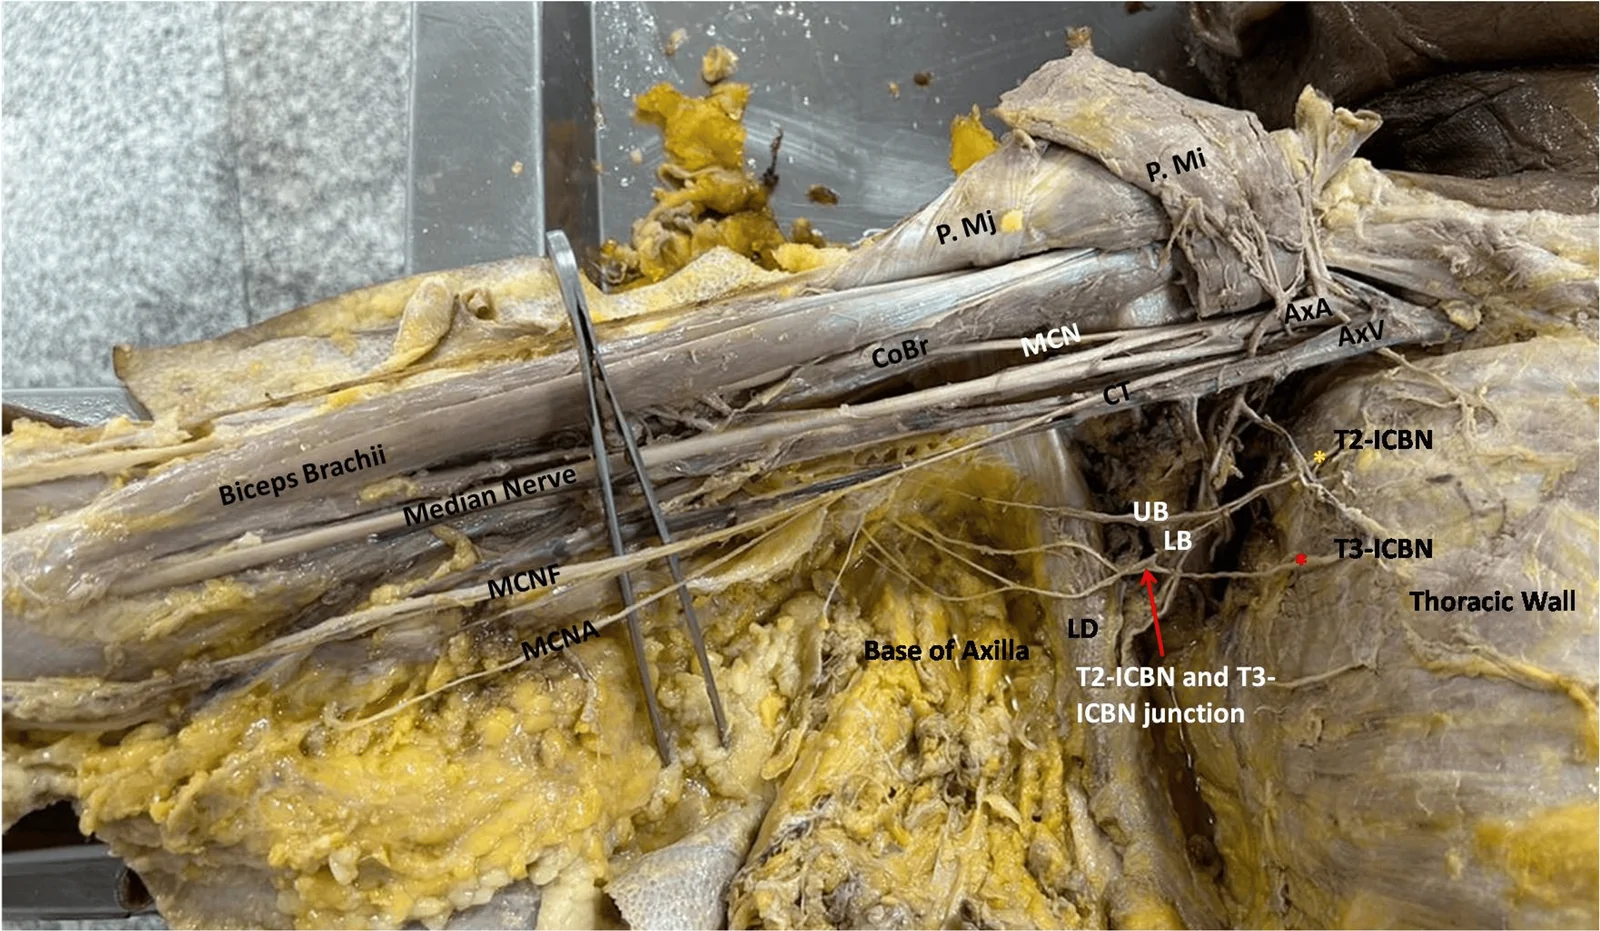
Dissected right axillary region showing the origin of the intercostobrachial nerve (ICBN) from the second intercostal space (T2-ICBN), its upper (UB) and lower (LB) branches, ICBN of the third intercostal space (T3-ICBN), the common trunk (CT) for the medial cutaneous of the arm (MCNA) and the medial cutaneous of the forearm (MCNF) P. Mi: pectoralis minor; P. Mj: pectoralis major; CoBr: coracobrachialis; LD: latissimus dorsi; AxA: axillary artery; AxV: axillary vein; MCN: musculocutaneous nerve; T2-ICBN: lateral cutaneous branch of the second intercostal nerve; T3-ICBN: lateral cutaneous branch of the third intercostal nerve; T2-ICBN and T3-ICBN junction shown by red arrow; T2-ICBN shown by yellow asterisk; T3-ICBN shown by red asterisk
